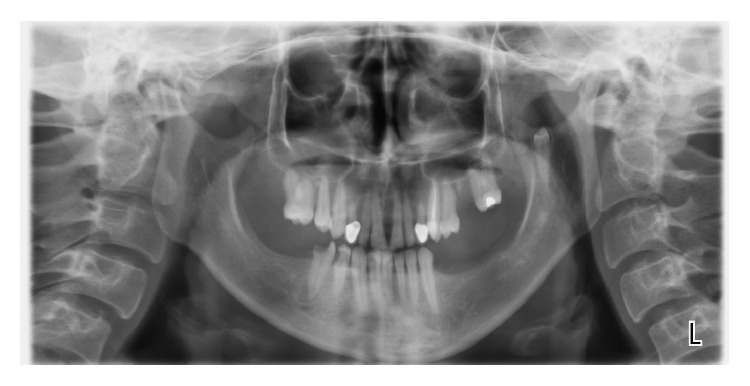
Panoramic radiograph showing an ectopic tooth located in the left mandibular ramus.
